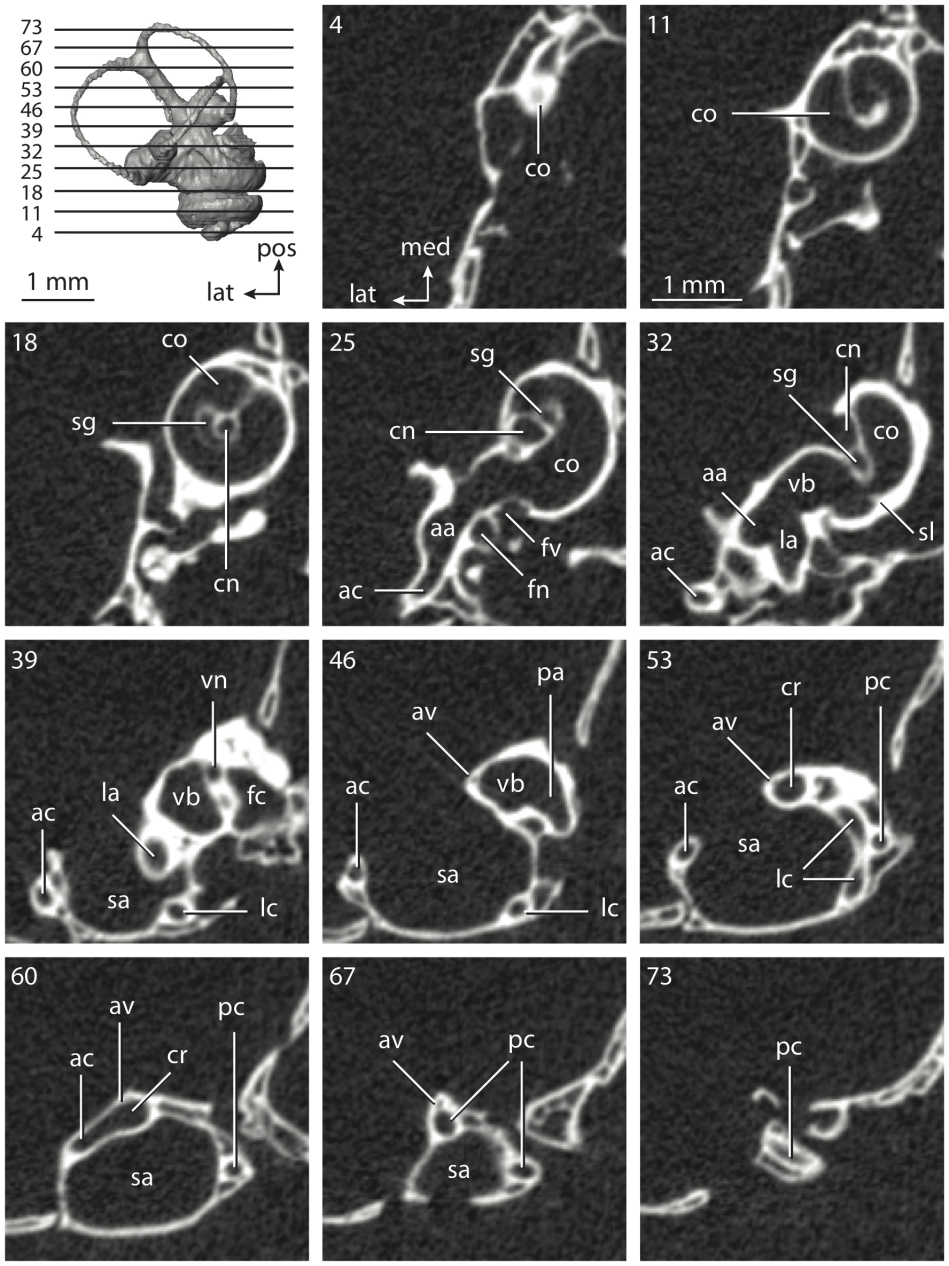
CT slices through ear region of*Mus musculus*. Abbreviations listed at the end of the Materials and Methods section.
